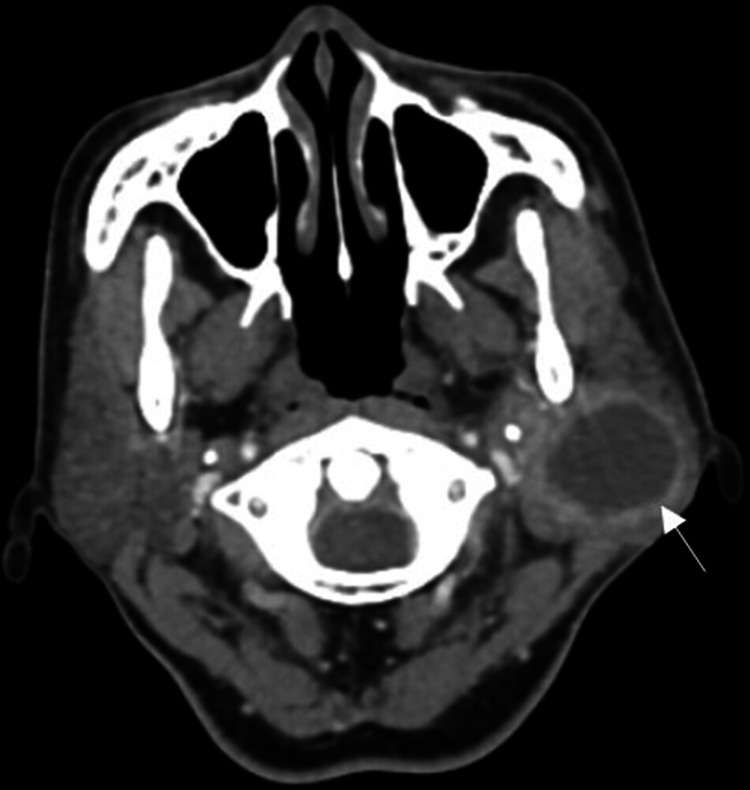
Axial CECT revealed a homogenous lesion in the left parotid gland with peripheral rim enhancement suggestive of an abscess (arrow). CECT - Contrast-enhanced computed tomography
